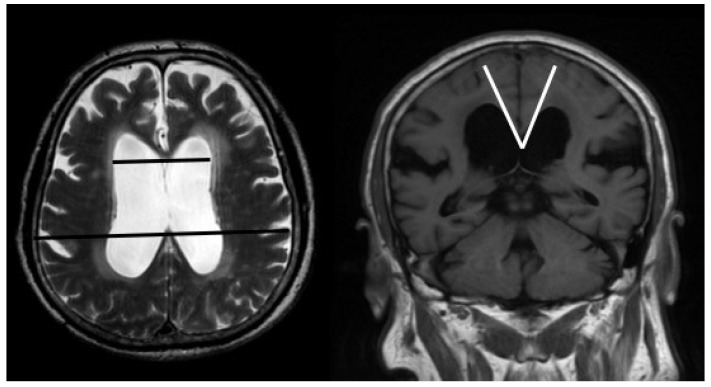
Pre-operative brain MRI (magnetic resonance imaging) of one representative patient with iNPH (idiopathic normal pressure hydrocephalus), who showed an increased Evans’ index (>0.3) and a sharp callosal angle (<90°).
